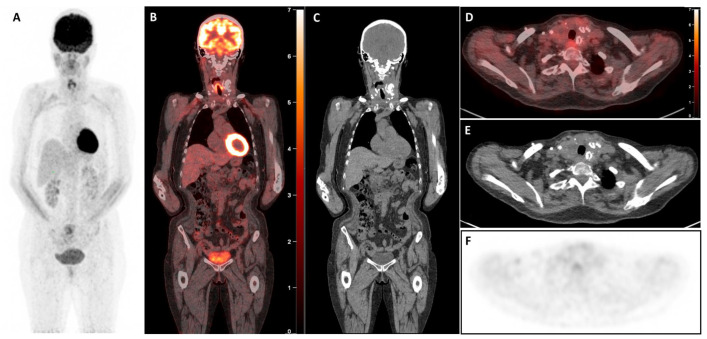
End-of-treatment [^18^F]FDG PET/CT showed normal tracer distribution on the whole-body MIP image (A), with no significant pathological uptake in the residual thyroid mass. The lesion demonstrated an SUVmax of 2.9, which was lower than hepatic uptake (SUVmax 3.3) but higher than the mediastinal blood pool (SUVmax 1.9), consistent with a complete metabolic response (Deauville score 3). (B,C) and (D–F) are coronal and axial images, respectively. B and D are PET and CT fused images.
